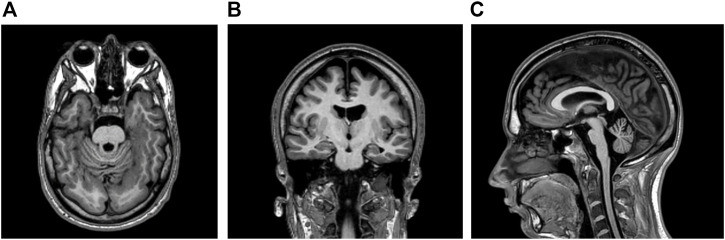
(A,B) Brain magnetic resonance imaging (MRI) showing bilateral polymicrogyria in temporal and occipital lobes (T1-weighted axial and coronal sequences respectively) (C) Atrophy in pons and cerebellum was detected in the T1-weighted sagittal sequence.
